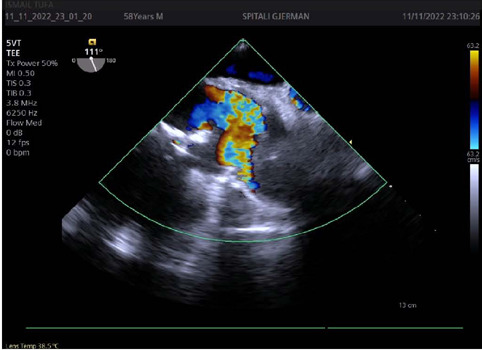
Transesophageal echocardiography showing accelerated flow because of mass
